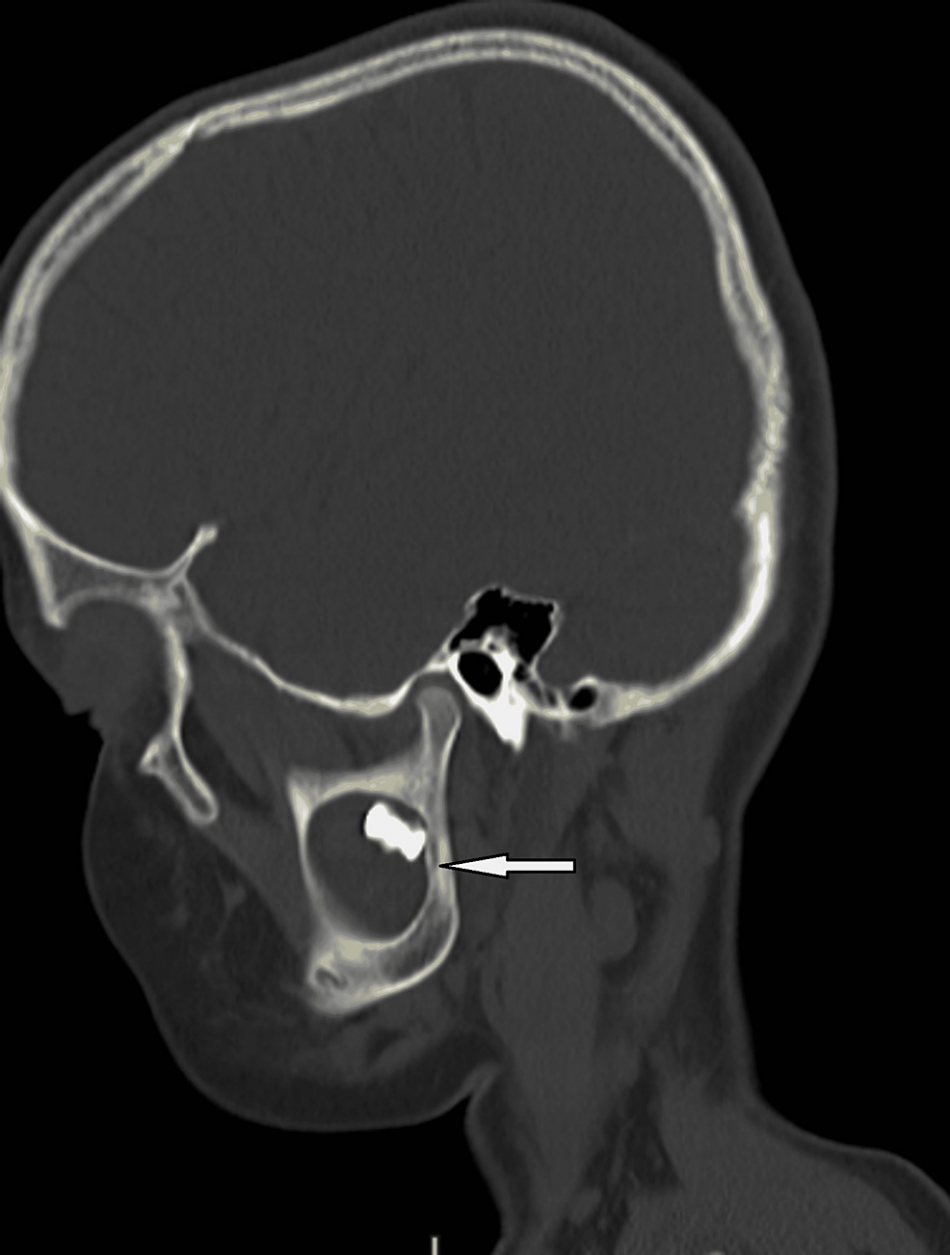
CT scan shows a lytic lesion with a tooth.
